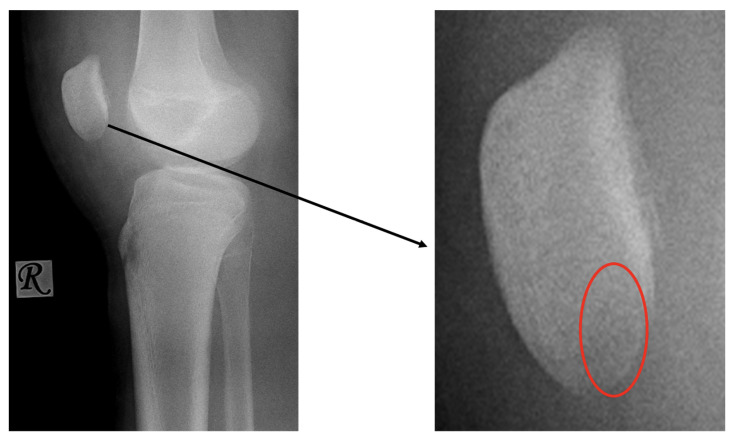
Postoperative X-ray presents the proper position of the osteochondral fragment of the patella (left image) on which the nails (red circle) are difficult to visualise.
